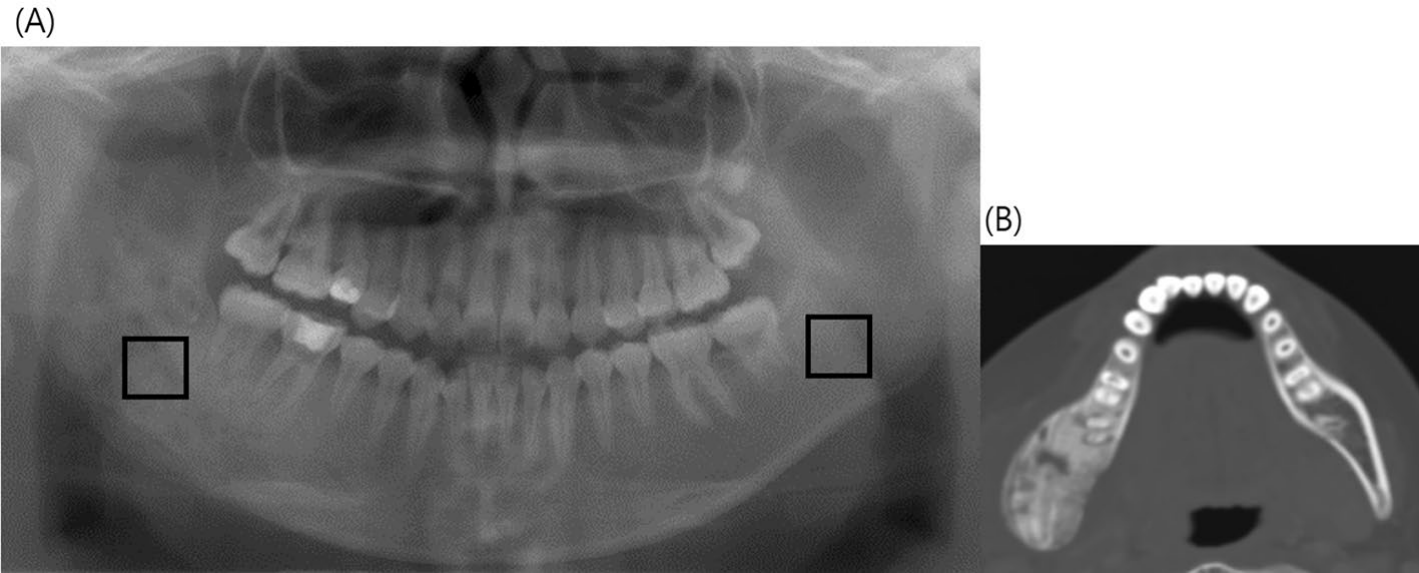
Panoramic radiograph of a 30-year-old woman with osteomyelitis (OM) on the right mandible. (A) A ROI was located at the center, or the representative area, of the lesion on a panoramic radiograph with reference to the corresponding CT image. The other ROI was placed in the corresponding normal trabecular bone on the left mandibular body. (B) CT image shows the OM lesion of the right posterior mandible more clearly.
